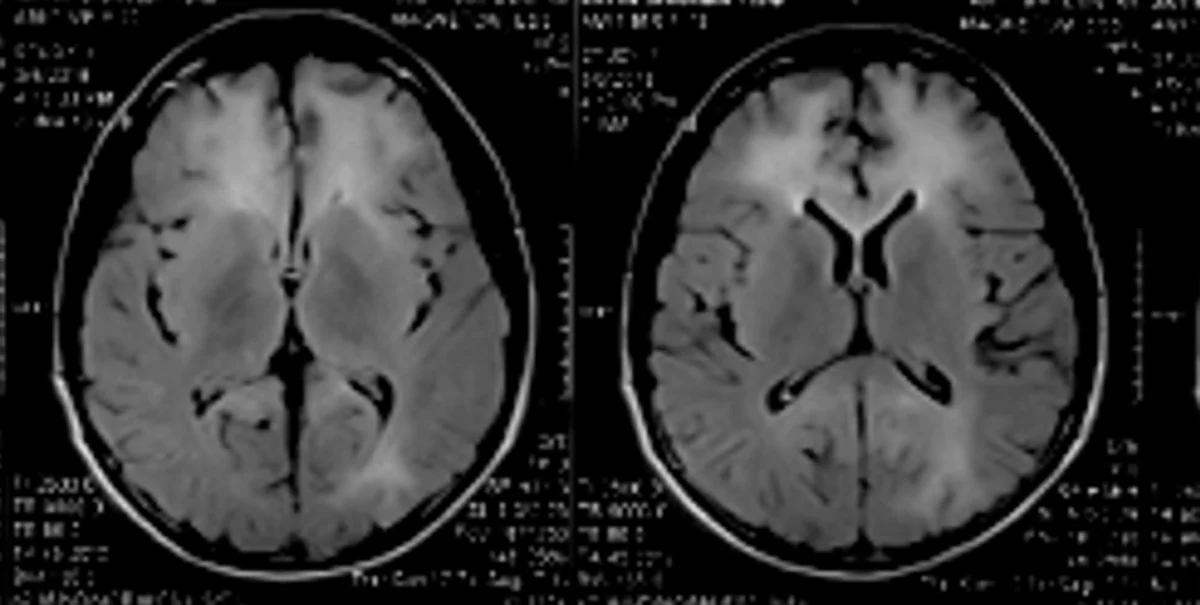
Brain MRI showing bilateral frontal grey and white matter lesions with involvement of the genu of the corpus callosum.
